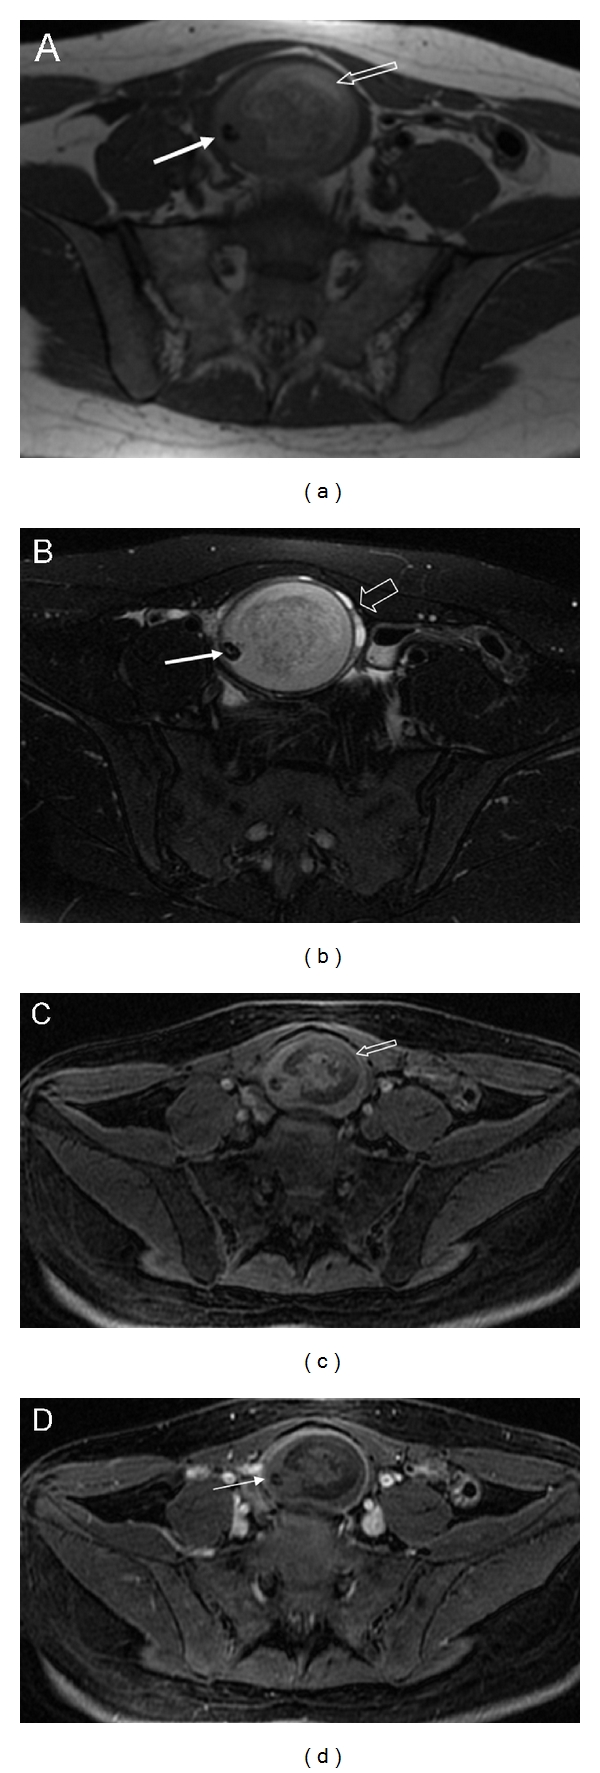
Dermoid cyst with small amount of fat. Axial T1- (a) and T2-weighted images with fat suppression (b) show a complex cystic round lesion with a hypointense nodule (a, b, white arrow) on the right side arising from the right ovary. Observe ovarian parenchyma with small follicles (b, void arrows). A linear T1 hyperintense portion is located on the upper part of the cyst (a, void arrow). The corresponding axial fat suppressed T1 weighted image shows the vanishing of the hyperintense part (c, arrow) confirming the presence of small amount of fat. 3D fat saturated T1-weighted Spoiled Gradient Echo image (d) demonstrates the poor enhancement of mural protrusion corresponding to Rokitansky nodule (arrow).
